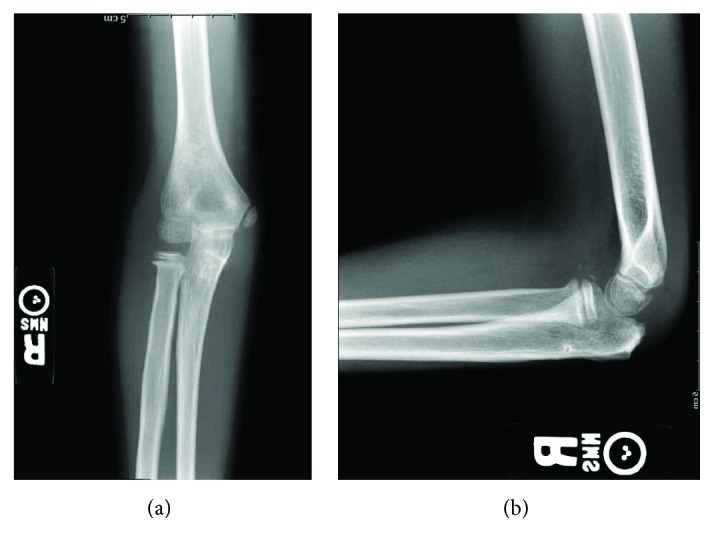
AP (a) and lateral (b) radiographs of the right elbow 7 months postoperatively demonstrating continued anatomic reduction of the ulnohumeral and radiocapitellar joints. The radial head and olecranon fractures are well healed. The patient's physes remain open.
